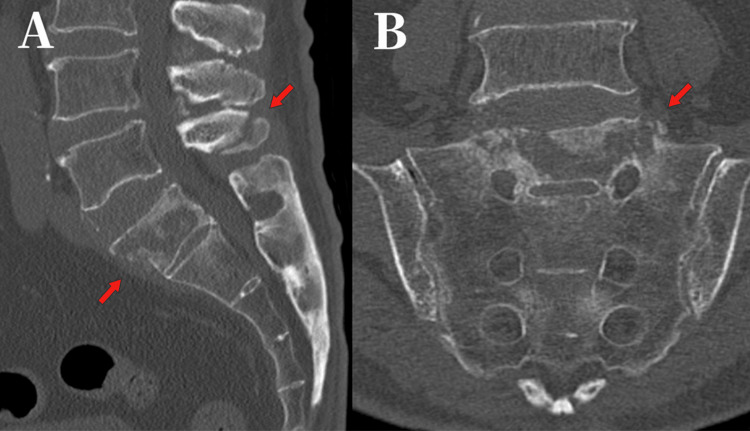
Sagittal and Coronal images. (A) Sagittal (B) Coronal views of the fracture site showing nonunion (18 weeks after injury). CT: computed tomography.
